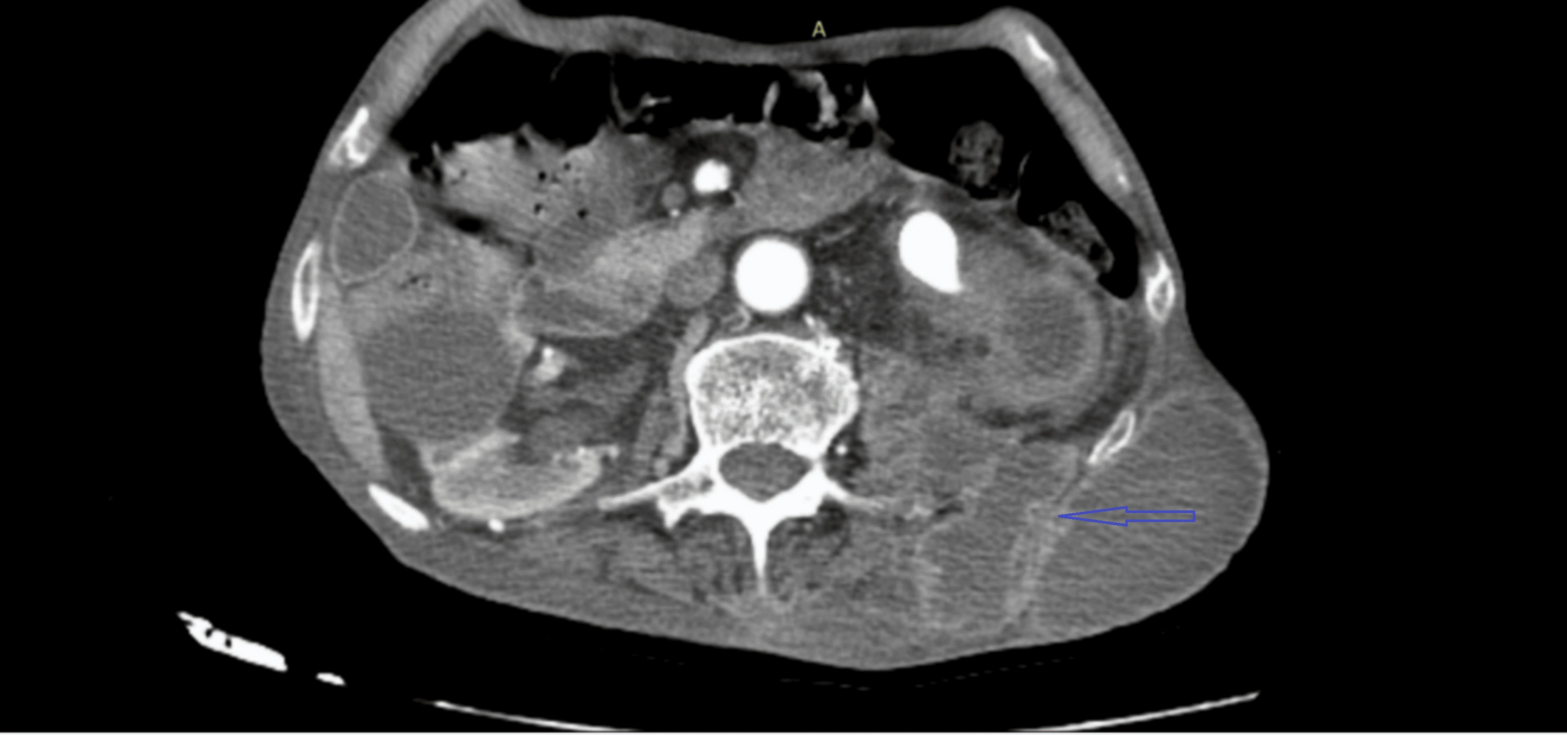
Axial image from the excretory phase of a contrast-enhanced abdominopelvic CT scan The image demonstrates left pyonephrosis caused by an obstructive stone in the renal pelvis, complicated by a retroperitoneal abscess with fistulization, leading to a subcutaneous lumbar collection in the same region indicated by the blue arrow.
